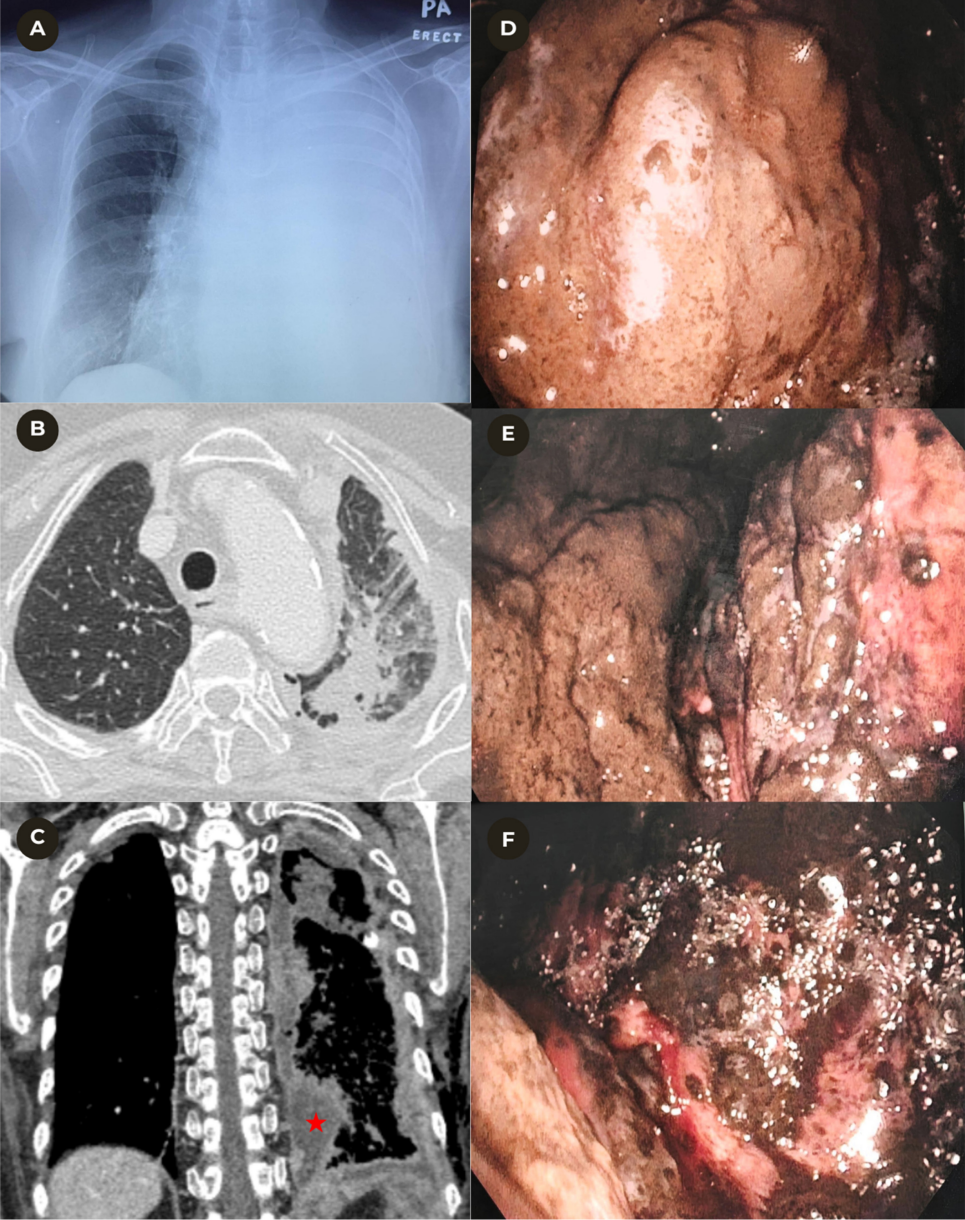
(A) Chest radiograph demonstrating a massive left‐sided pleural effusion. (B) Axial CT thorax (lung window) showing a spiculated 3.3 × 2.4 × 2.6 cm mass in the left apicoposterior segment with interlobular septal thickening and adjacent ground‐glass opacities. (C) Coronal CT thorax (mediastinal window) demonstrating lobulated, enhancing pleural nodules involving the mediastinal and parietal pleura with associated loculated pleural effusion (red star). (D) Thoracoscopic view of the anterior parietal pleura showing nodular pigmented thickening. (E) Thoracoscopic view of the visceral pleura demonstrating nodular pigmented lesions. (F) Thoracoscopic view of the diaphragmatic pleura showing multiple nodular pigmented lesions.
